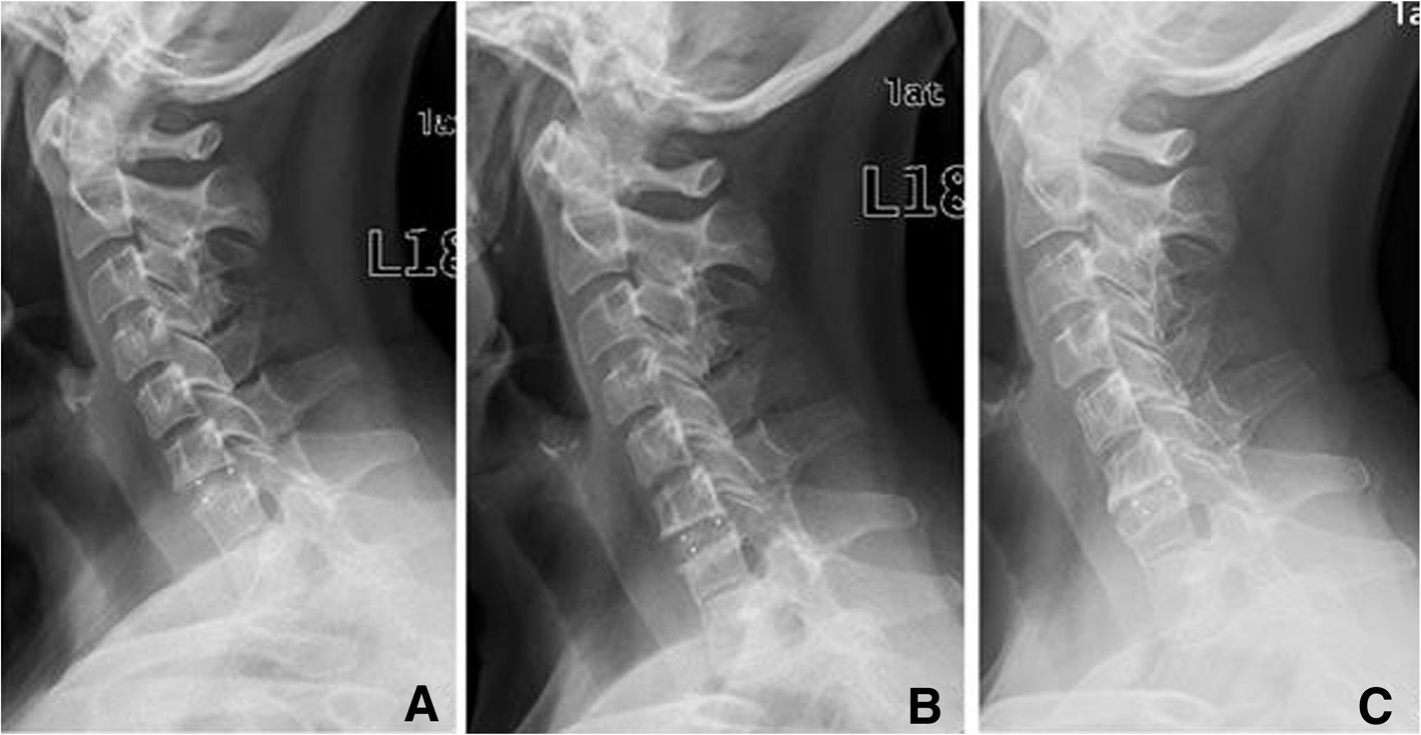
Cervical spine (female, 59 years) with spinal stenosis and advanced disc degeneration in C6/7 and spontaneous block vertebra formation in C7/Th1 treated with a cervical cage in C6/7 – X-rays taken A immediately postoperatively, B 1 year after surgery with initial ventral subsidence of the cage into the superior endplate of C7, and C 2.5 years postoperatively with advanced ventral subsidence and osseous bridging.
